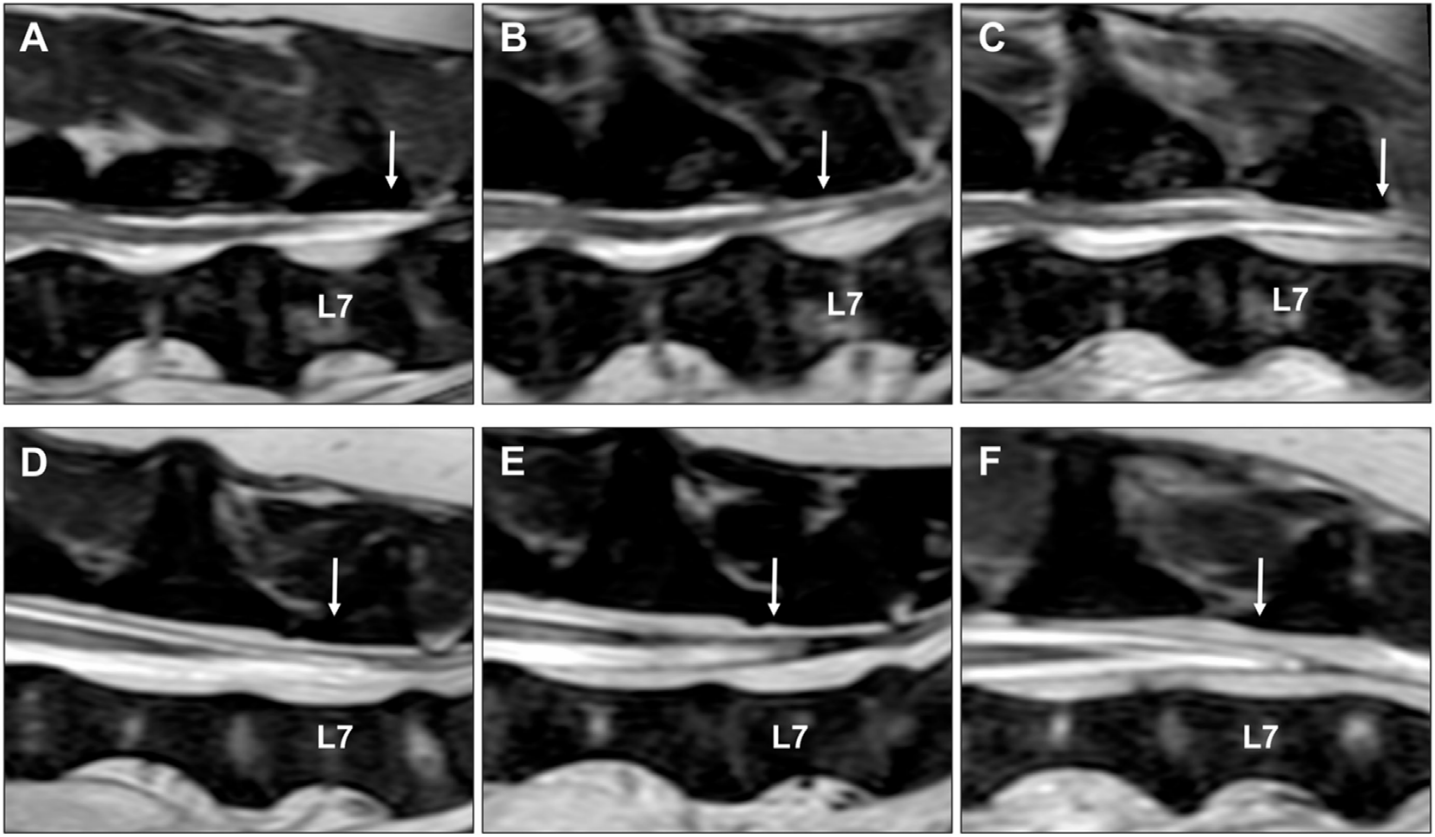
Sagittal T2-weighted images of an English Cocker spaniel with thoracolumbar intervertebral disk extrusion with the hips in neutral (A), extended (B), and flexed (C) positions. (A) The conus medullaris (arrow) terminates at the caudal aspect of the L7 vertebral body. (B) The conus medullaris (arrow) is displaced in a cranial direction when the hips are extended and terminates now halfway the L7 vertebral body. (C) The conus medullaris (arrow) is displaced in a caudal direction when the hips are flexed and terminates now at the level of the L7–S1 intervertebral disk space. Sagittal T2-weighted images of an English Cocker spaniel with tethered cord syndrome with a tight filum terminale with the hips in neutral (D), extended (E), and flexed (F) positions. The conus medullaris (arrow) terminates halfway the L7 vertebral body in all three positions, and only minimal displacement is seen between different positions.
